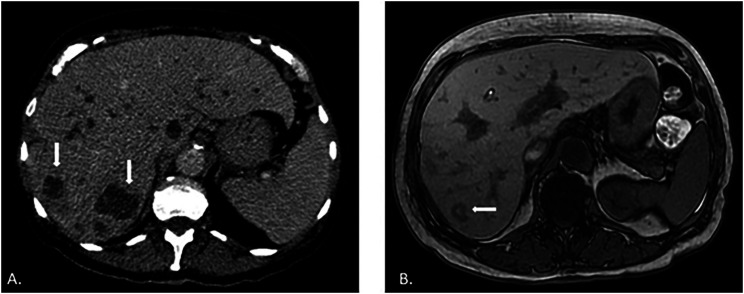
(A) Abdominal CT performed before treatment, showing multiple lobulated expansive formations in the right lobe of the liver, the largest measuring 3.4 cm; (B) Abdominal MRI performed after treatment, showing a nodular image with hyposignal on T1, located in segment VII of the liver, measuring 1.8 cm (smaller compared to the previous tomographic image).
